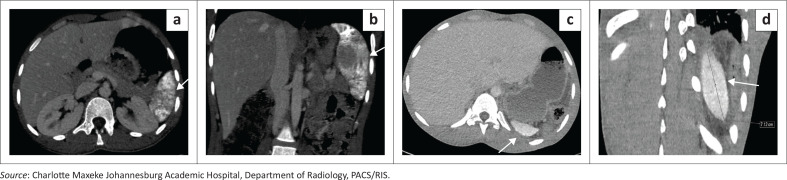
Splenic findings in sickle cell disease in a 24-year-old male presenting with jaundice and anaemia and in a separate patient with known sickle cell disease presenting during a chest crisis. (a, b) Axial contrast-enhanced CT images demonstrate coarse patchy calcifications within a normal-sized spleen (arrows), reflecting repeated splenic infarctions. (c, d) Axial contrast-enhanced CT images in a different patient demonstrate a diffusely hyperdense heavily calcified small spleen consistent with autosplenectomy (arrows).
